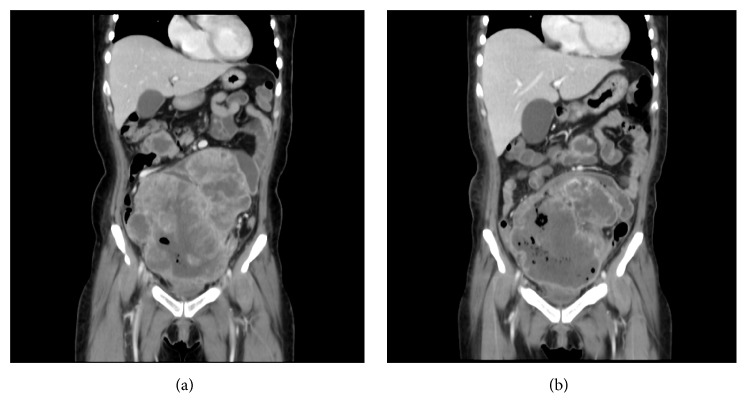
(a) Enhanced abdominal computed tomography (coronal section) reveals the remaining tumor is approximately 20.3 cm in diameter before irradiation. (b) After radiation therapy, the tumor size decreased to 15.3 cm.
